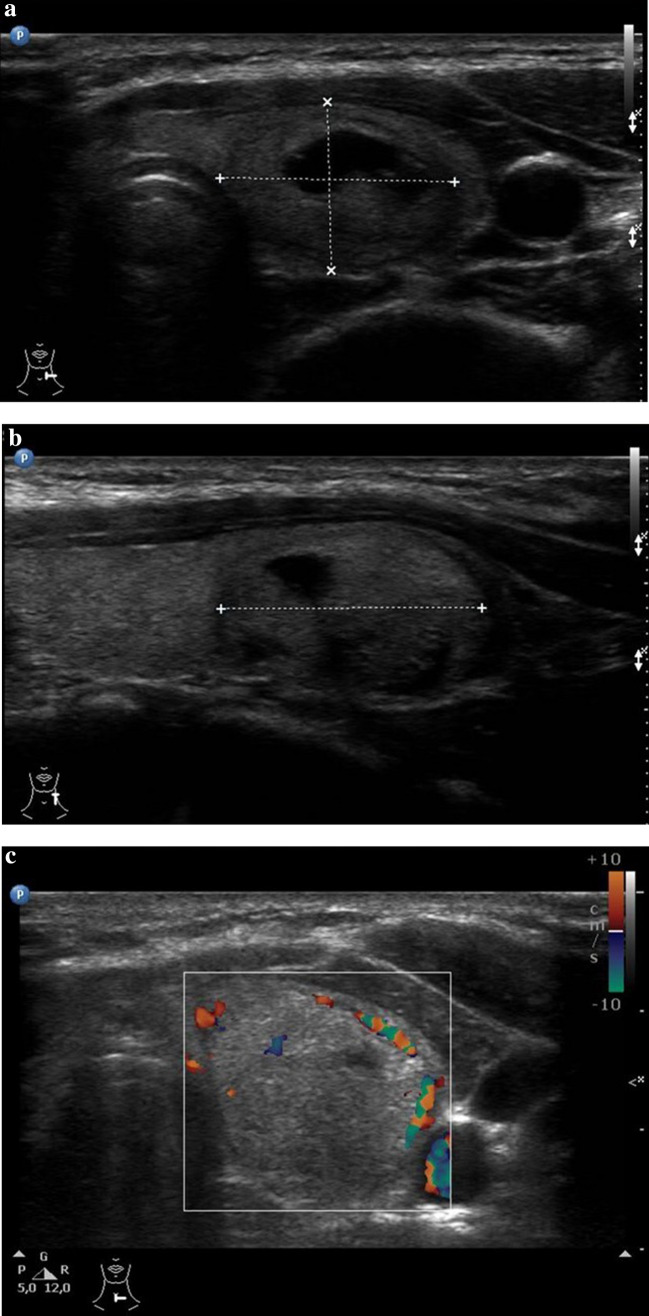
Conventional US and color Doppler US images of benign thyroid lesions. a B-mode US image in a transversal plane of the left thyroid lobe shows a partially fluid filled regressive lesion with a mixed echo structure and hypoechoic borders in a 5-year-old girl with nodular goiter. b B-mode US image in a longitudinal plane of the left thyroid lobe shows a slightly hyperechoic, partially fluid filled regressive lesion with hypoechoic borders in a 5-year-old girl with nodular goiter. c Color Doppler US image in a transverse plane shows little vascular flow in a thyroid lesion in a 5-year-old girl with nodular goiter
